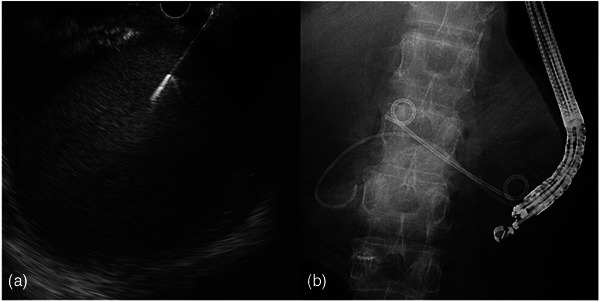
(a) An infected liver cyst was punctured from the stomach using an echoendoscope. (b ) Endoscopic ultrasound‐guided drainage was performed for the liver cyst, and internal and external drainage were placed.
